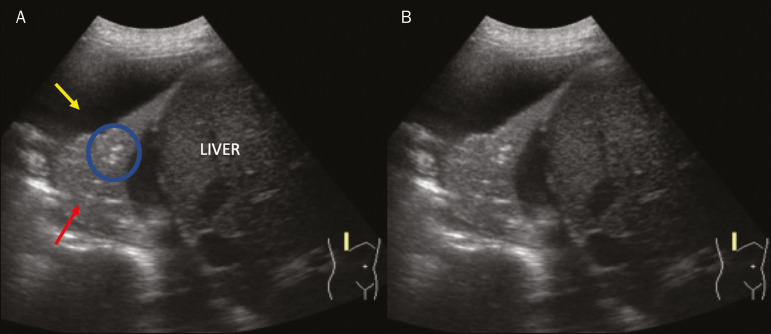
A,B: Ultrasound view of the lower third of the right hemithorax. Atelectasis (red arrow) and pleural effusion (yellow arrow). Note the volumetric reduction of the pulmonary segment assessed and the presence of static air bronchogram (circle). During respiratory incursions, there is no change in its characteristics and in the Doppler phenomenon, which would be present in the dynamic bronchograms of consolidations.
